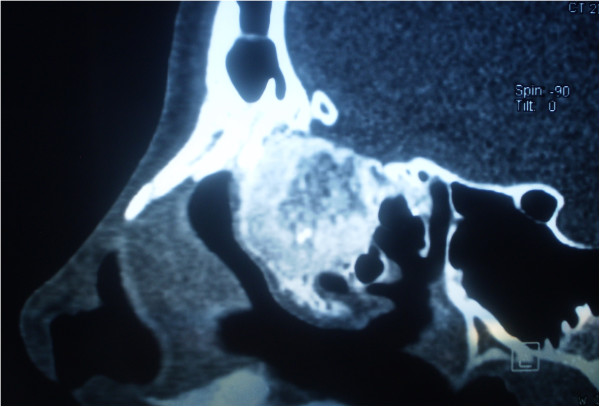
Sagittal multislice computed tomography showed a clearly delineated, heterogeneous (hyperechoic) tumor growth with inhomogeneous central portion and marginal mineralization.
